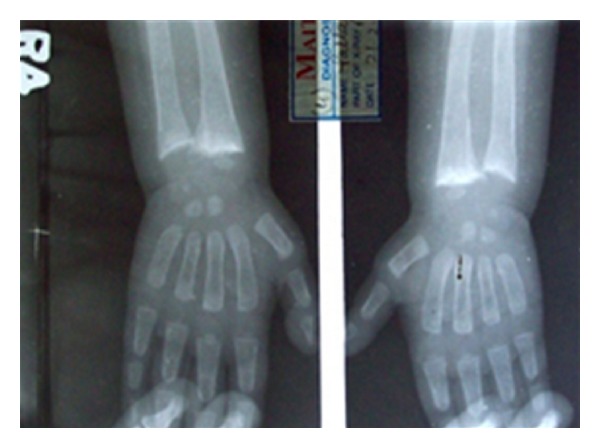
Showing wrist X-ray with features of active rickets.
